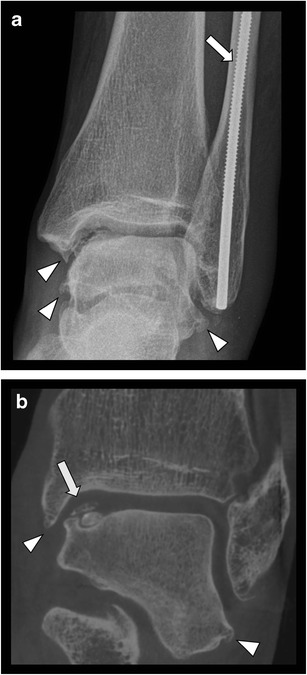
Posttraumatic unstable osteochondral lesion of the talus and massive degenerative changes of the ankle in a 66-year-old female. a. CR (AP view) demonstrating the presence of metallic screw within the fibula (arrow). Note irregular articular surface of talar dome and advanced degenerative changes with osteophytes formation (arrowheads). b. CBCT coronal reformatted image better shows the presence and extent of an unstable osteochondral lesion (arrow) and osteophytes (arrowheads). There is no metal artifact from the screw in the fibula
